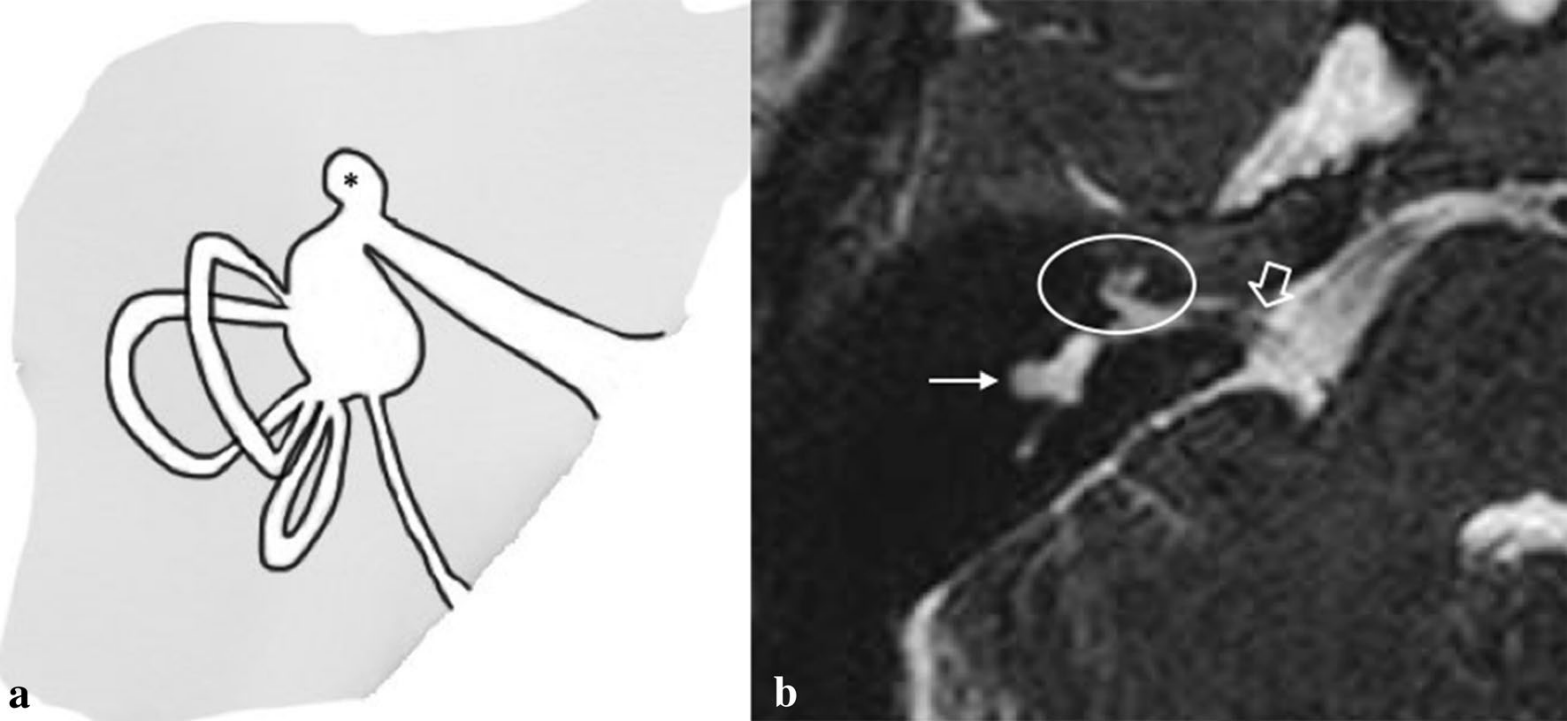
Cochlear hypoplasia. a The chart shows type I cochlear aplasia. b A 16-year-male with right SN deafness from birth. Axial MRI 3D FIESTA sequence obtained at IAC level shows cochlear hypoplasia (circled), stenosis of the right IAC (open arrow) with cochlear nerve hypoplasia, and LSCC dysplasia (white arrow)
